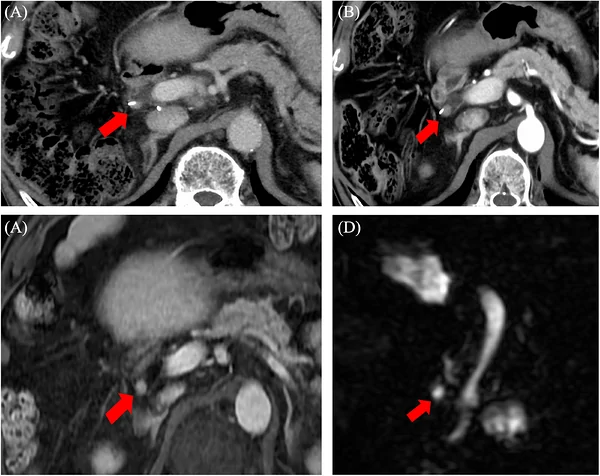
Radiological findings of the lesion. (A) Contrast‐enhanced computed tomography (CT) obtained 4 years before presentation showed a small enhancing nodule at the stump of the remnant cystic duct (red arrow). (B) Arterial‐phase contrast‐enhanced CT demonstrated an 8‐mm enhancing mass at the stump of the remnant cystic duct (red arrow). (C) Arterial‐phase gadolinium ethoxybenzyl diethylenetriamine pentaacetic acid‐enhanced magnetic resonance imaging (EOB‐MRI) showed a well‐defined enhancing mass at the stump of the remnant cystic duct (red arrow). (D) Coronal magnetic resonance cholangiopancreatography (MRCP) demonstrated the remnant cystic duct (red arrow), corresponding to the location of the lesion. No biliary stricture or upstream bile duct dilatation was observed.
